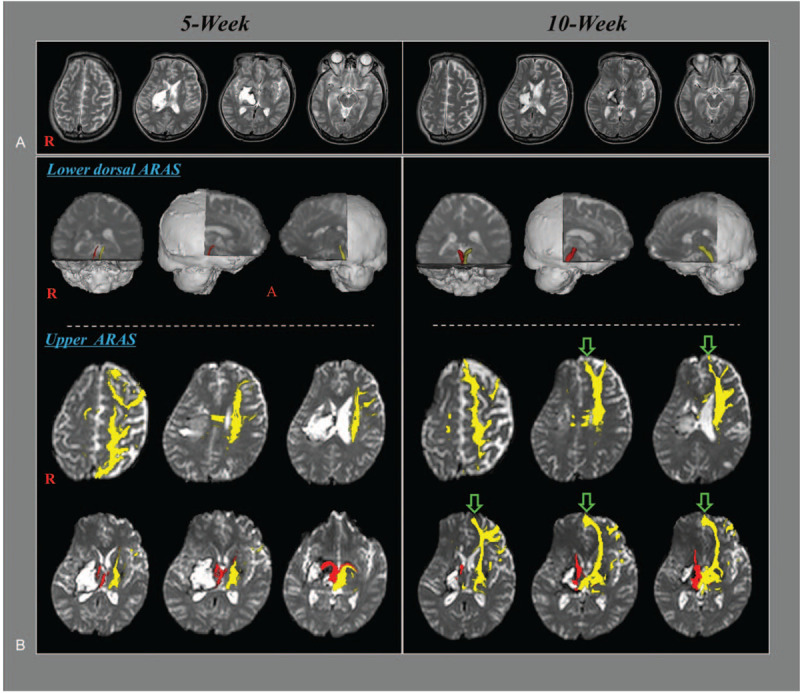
(A) Brain magnetic resonance images at 5 and 10 weeks after onset show leukomalactic lesions in the right basal ganglia and thalamus. (B) Results of diffusion tensor tractography (DTT) of the ascending reticular activating system (ARAS). On the 10-week DTT, thickening of the lower dorsal ARAS is observed on both sides when compared with the 5-week DTT. Decreased neural connectivity to the left prefrontal cortex is observed on the 5-week DTT whereas the decreased neural connectivity to the left prefrontal cortex is increased on the 10-week DTT, especially the medial prefrontal cortex (green arrows).
